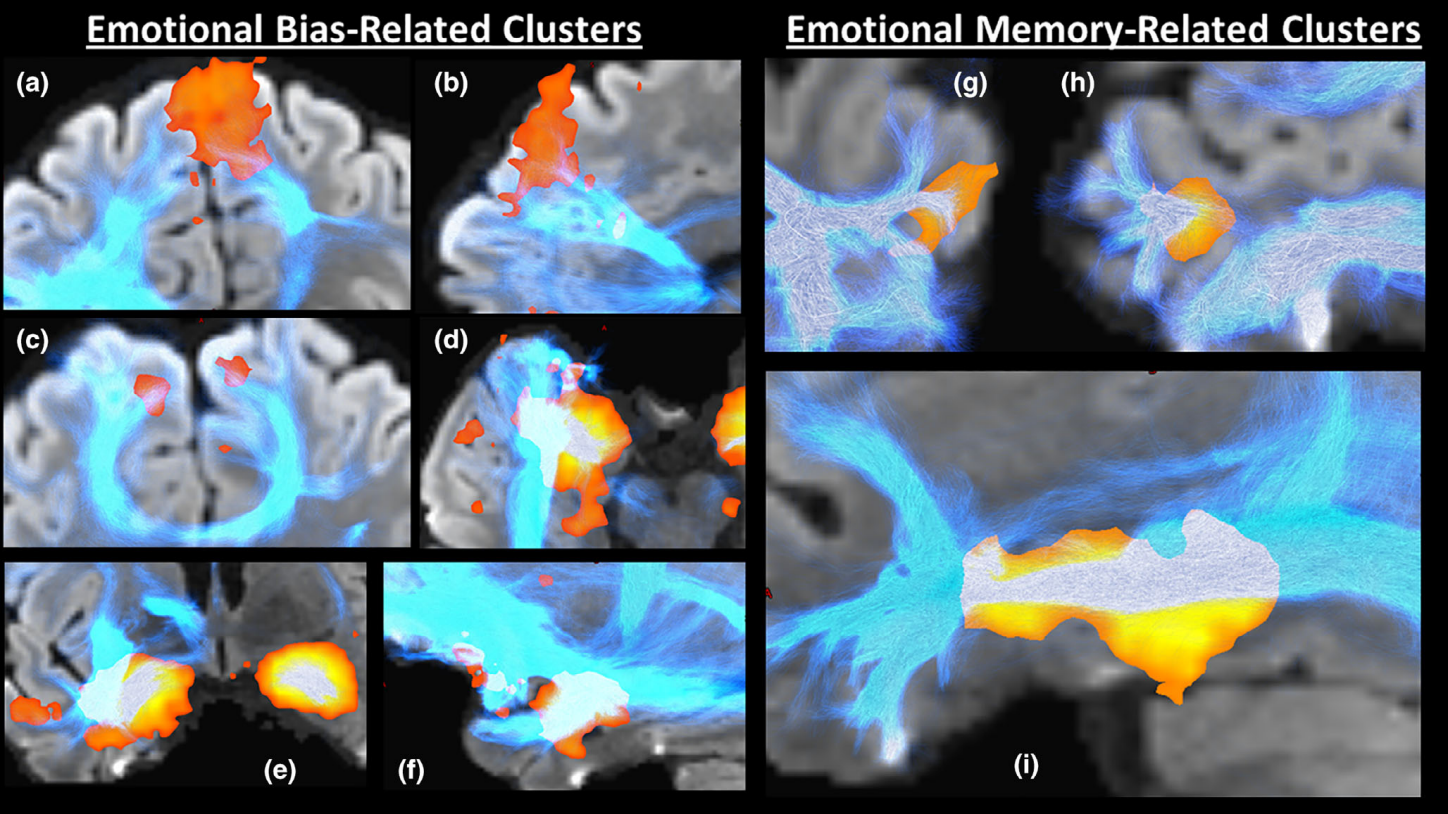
Probabilistic diffusion tractography of the identified tracts. In blue are the probabilistic streamlines generated using the significant clusters as a seed region. The orange regions are gathered from automated NeuroSynth meta‐analyses of functional MRI studies of emotional memory or emotional bias. The subfigures are as follows: (a, b, c) medial prefrontal cortex emotional bias‐related cluster with intersecting tracks bilaterally mostly via the corpus callosum superior genu in order of coronal, sagittal and axial views, (d, e, f) hippocampal emotional bias clusters intersected in the right hemisphere by tracks primarily from the uncinate and inferior longitudinal fasciculi in order of axial, coronal and sagittal views, (g, h) left middle temporal gyrus emotional memory cluster intersected by tracks from the inferior longitudinal fasciculus from axial and sagittal views, and (i) left hippocampus‐amygdala emotional memory cluster intersected by inferior longitudinal fasciculus tracks in a sagittal view
